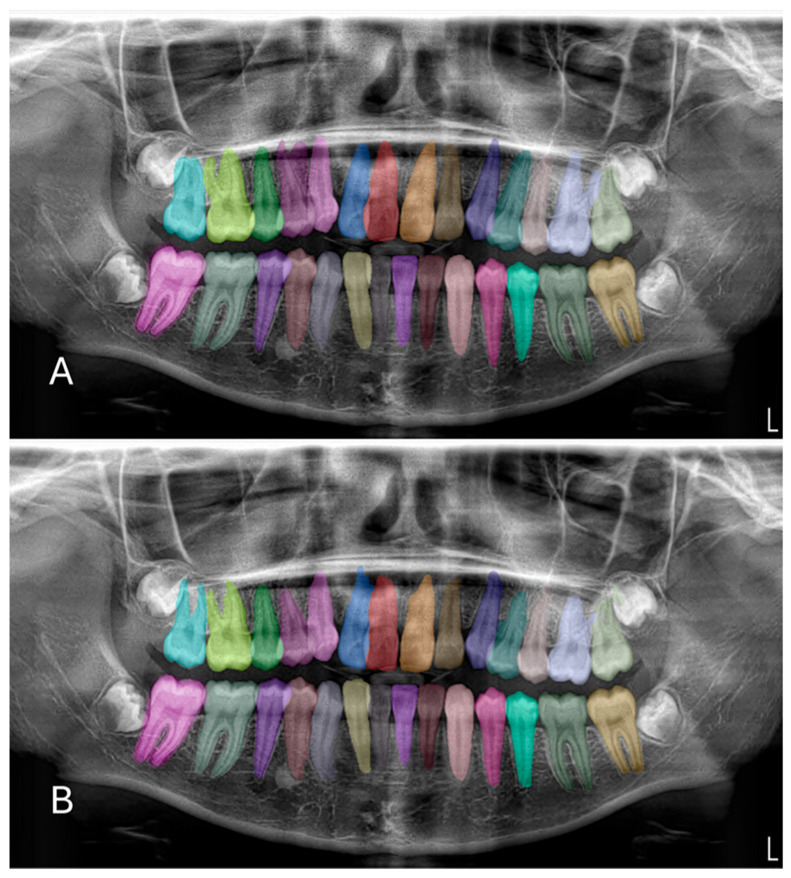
Example images demonstrating the performance for segmentation of permanent teeth on panoramic radiographs between manual annotation by researchers and U-Net based artificial intelligence (AI)-predicted label masks. (A) Manual segmentation performed by clinicians. (B) Automatic segmentation by the model. In the segmentation figures, each tooth is represented with a distinct colour to visually differentiate regions; however, the model treated all teeth as unified segmentation classes during training.
